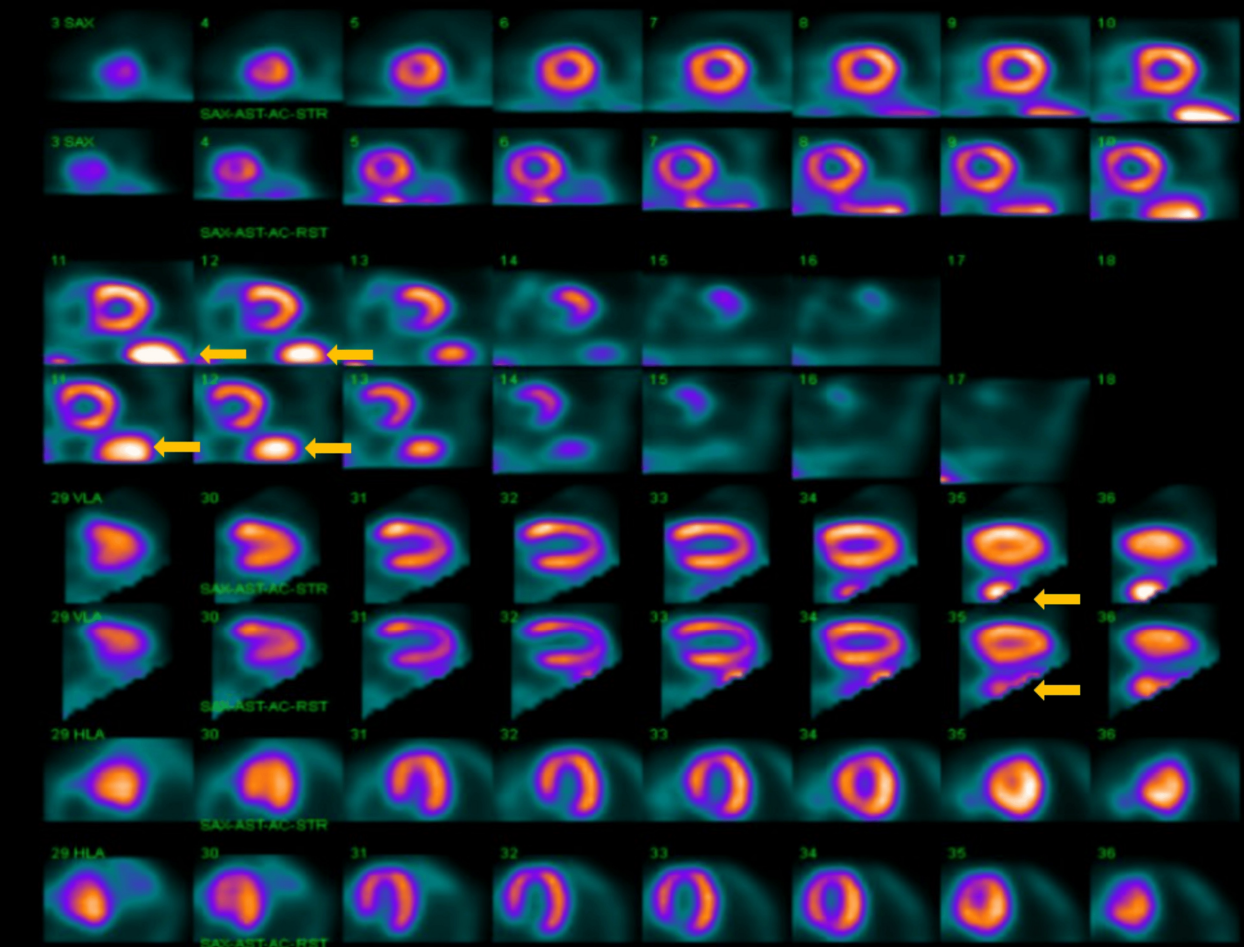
Single-photon emission computed tomography (SPECT) imaging with technetium showing no perfusion abnormalities, but visceral artifact is noted (yellow arrows) There are four sets of row, where the top row indicates perfusion in stressed heart, while lower row indicates perfusion in a resting heart. The first two rows are short axis images coming form the apex of the heart to the base, the third row is the vertical long axis view, and the fourth row is the horizontal long axis view.
